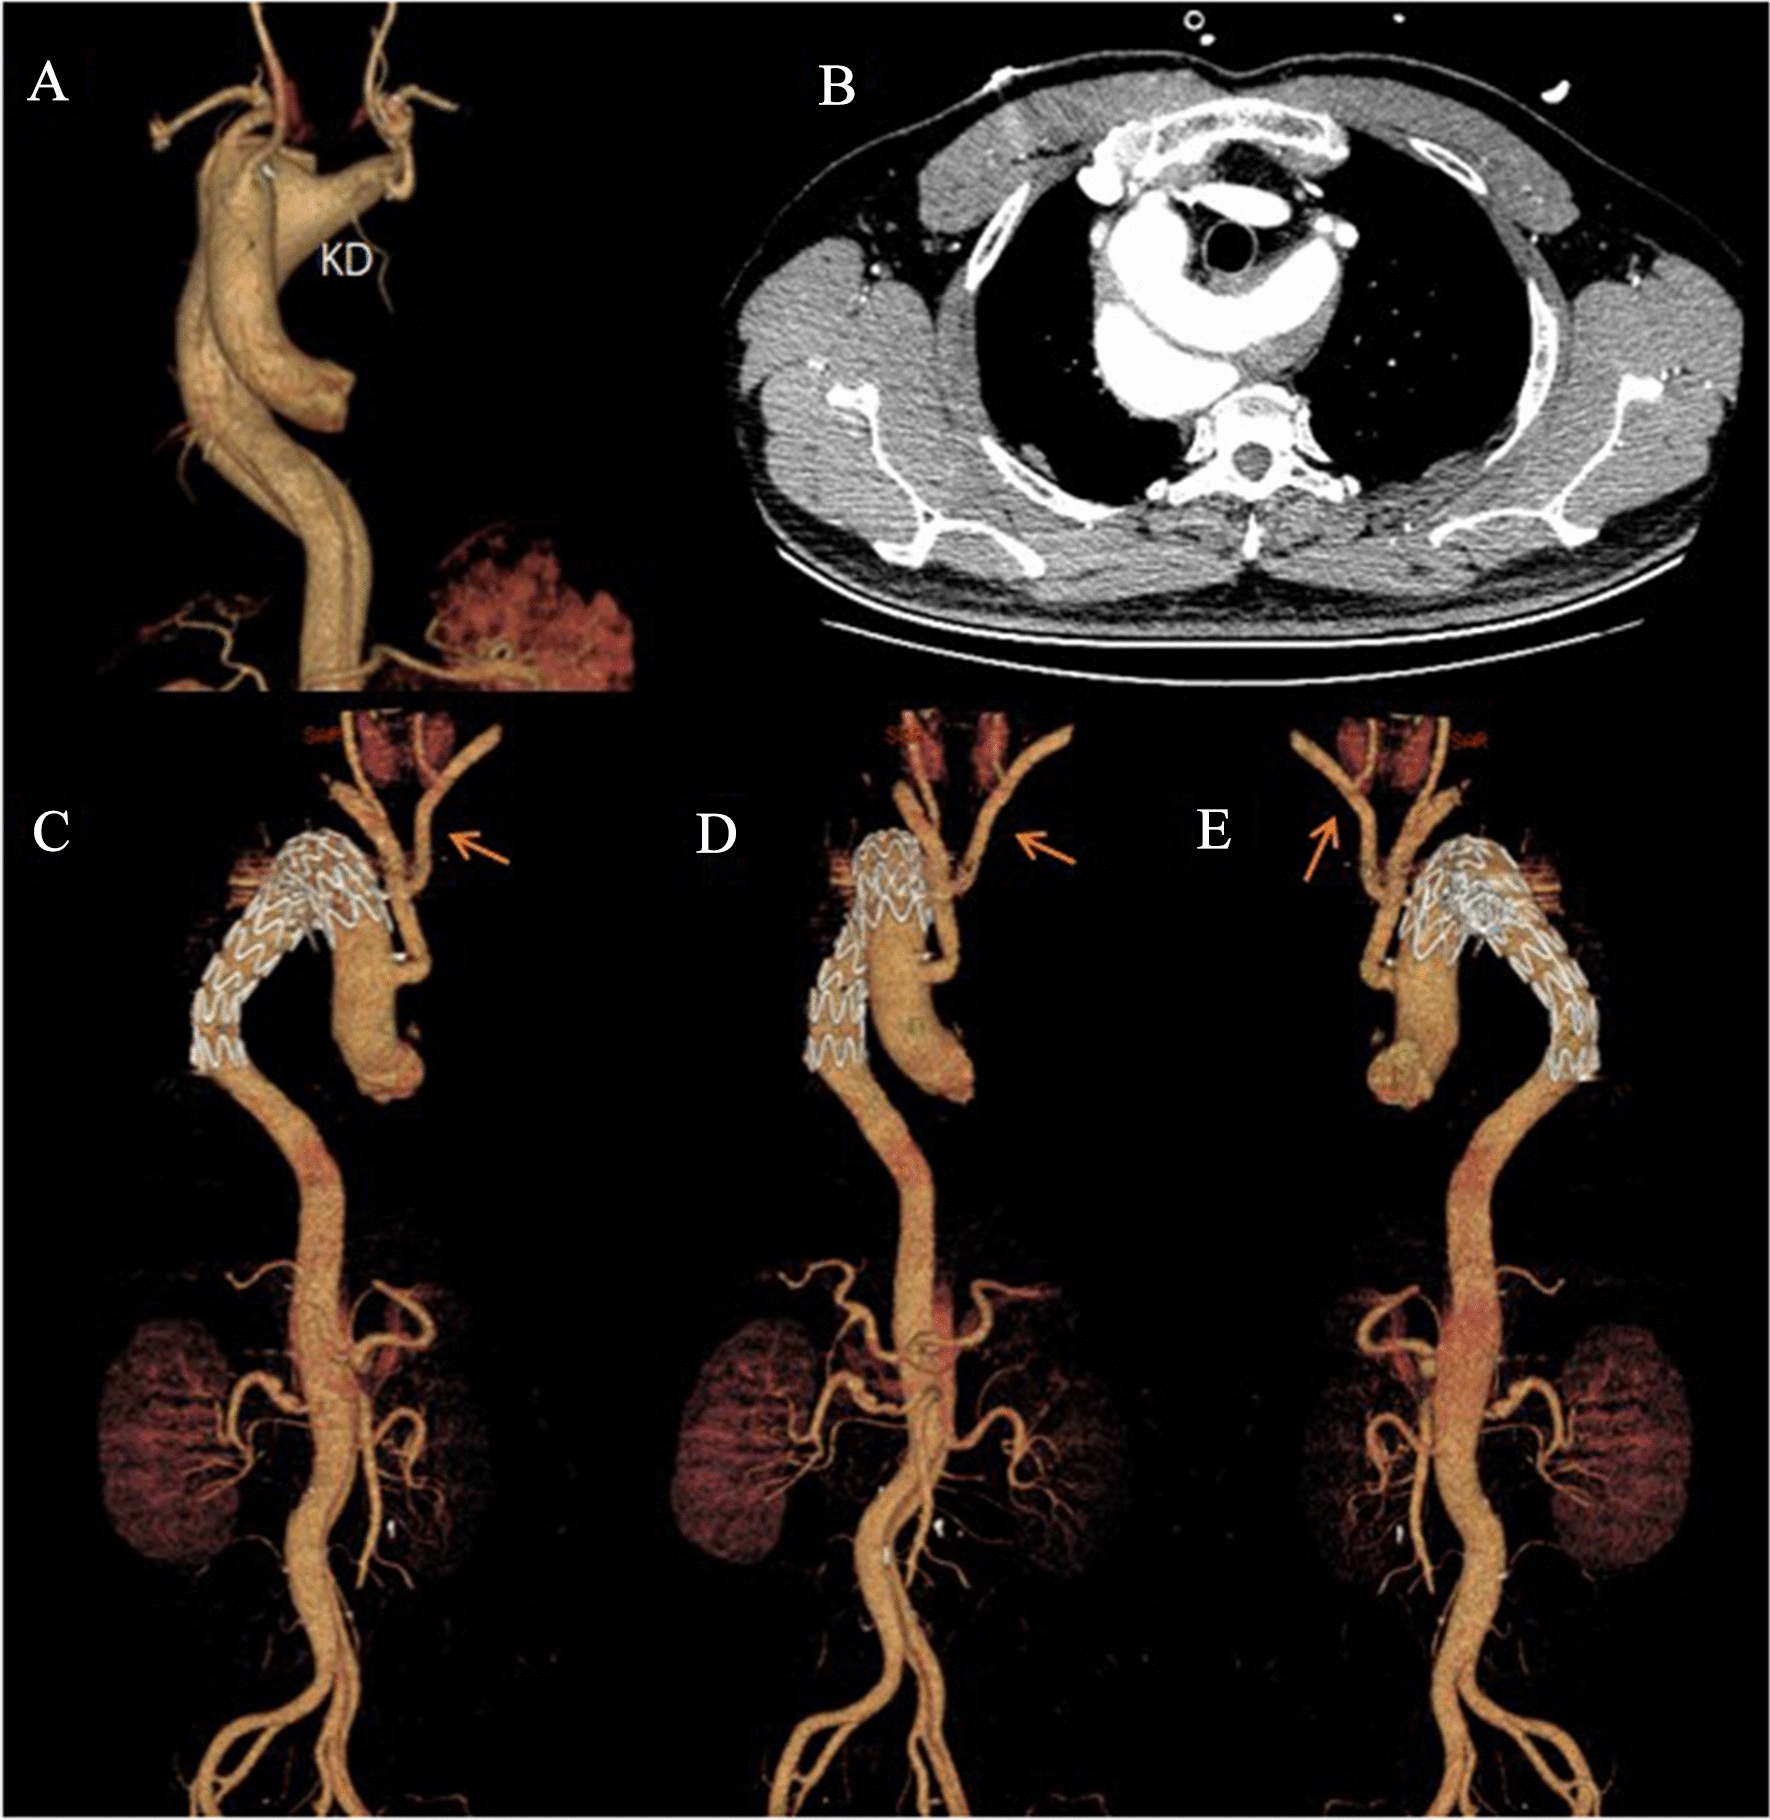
Hybrid procedure combining open arch repair and endovascular intervention. 3D reconstruction and preoperative CT scan of a right-sided arch with a Stanford B type aortic dissection involving the KD with aberrant LSA (A, B); reconstruction image of postoperative computed tomographic angiogram shown in different direction (C–E) and anastomosing branched vessels by Y-shape graft (orange arrow)
